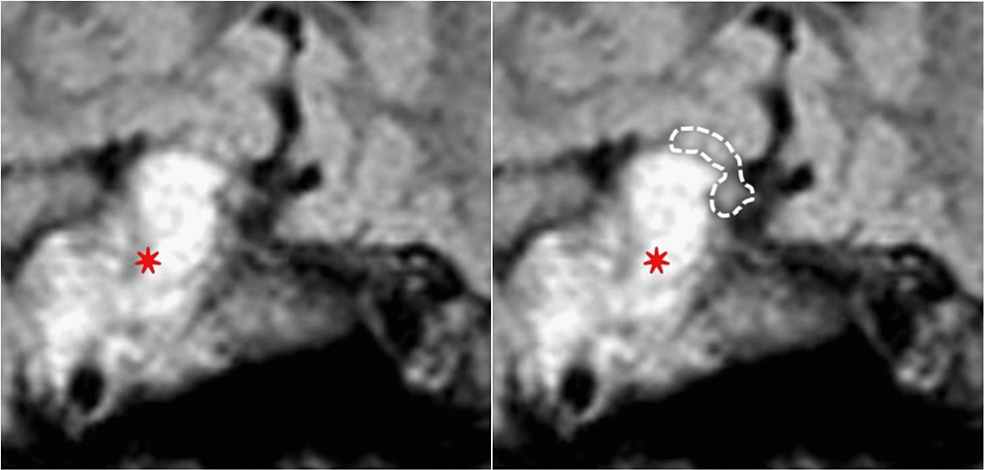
Brain MRI: High magnification view in the chiasmatic region showing the tumor (red star) displacing the chiasm (dotted line on the right) upward and asymmetrically compressing it on the right.
